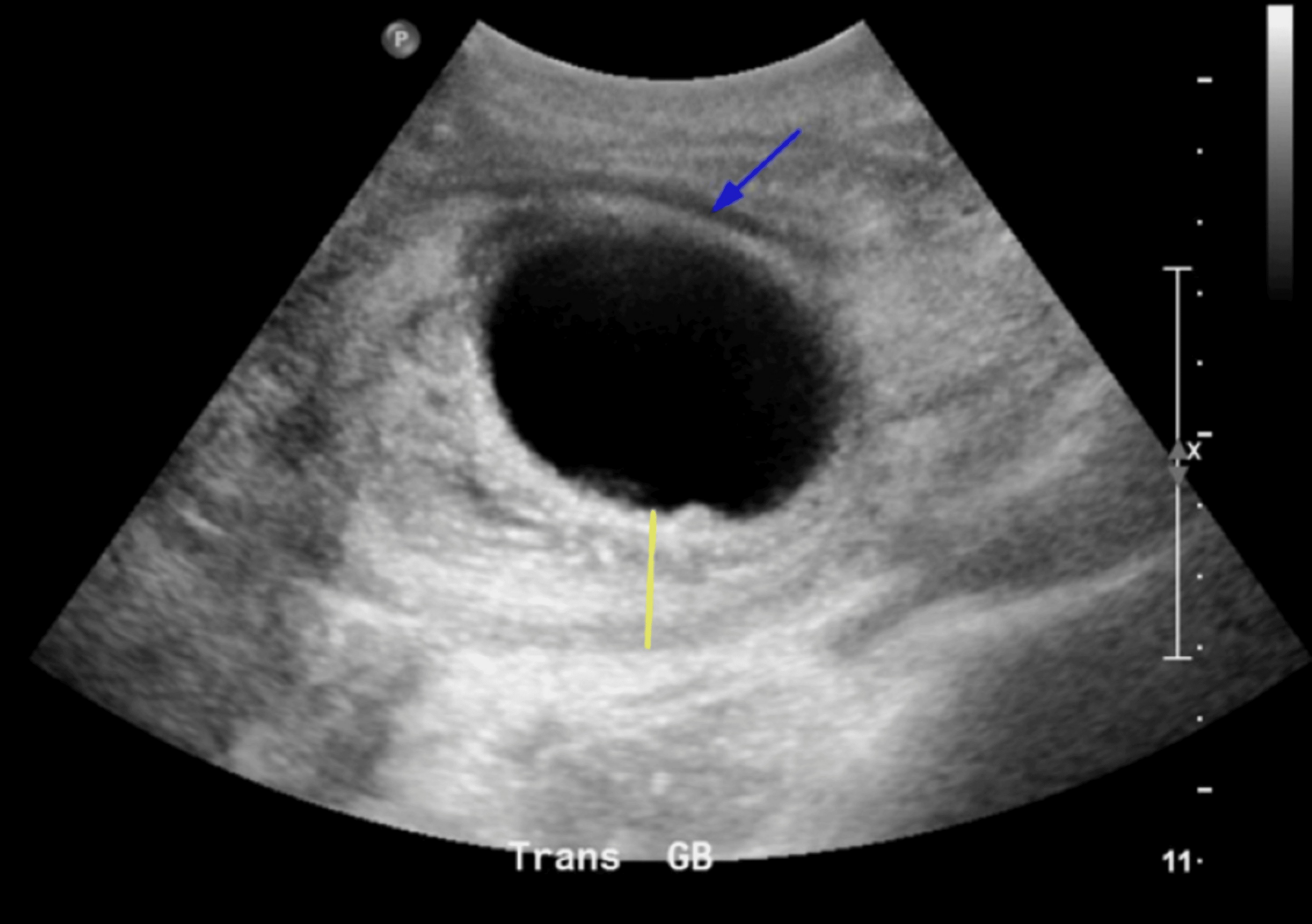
Transverse Ultrasound of the Gallbladder Demonstrating Wall Thickening and Pericholecystic Fluid Transverse ultrasound of the gallbladder showing marked distention, previously measured at 11 cm (not to scale in this image). Wall thickening is demonstrated (yellow line, not to scale), and pericholecystic fluid is noted (blue arrow), consistent with hemorrhagic cholecystitis.
